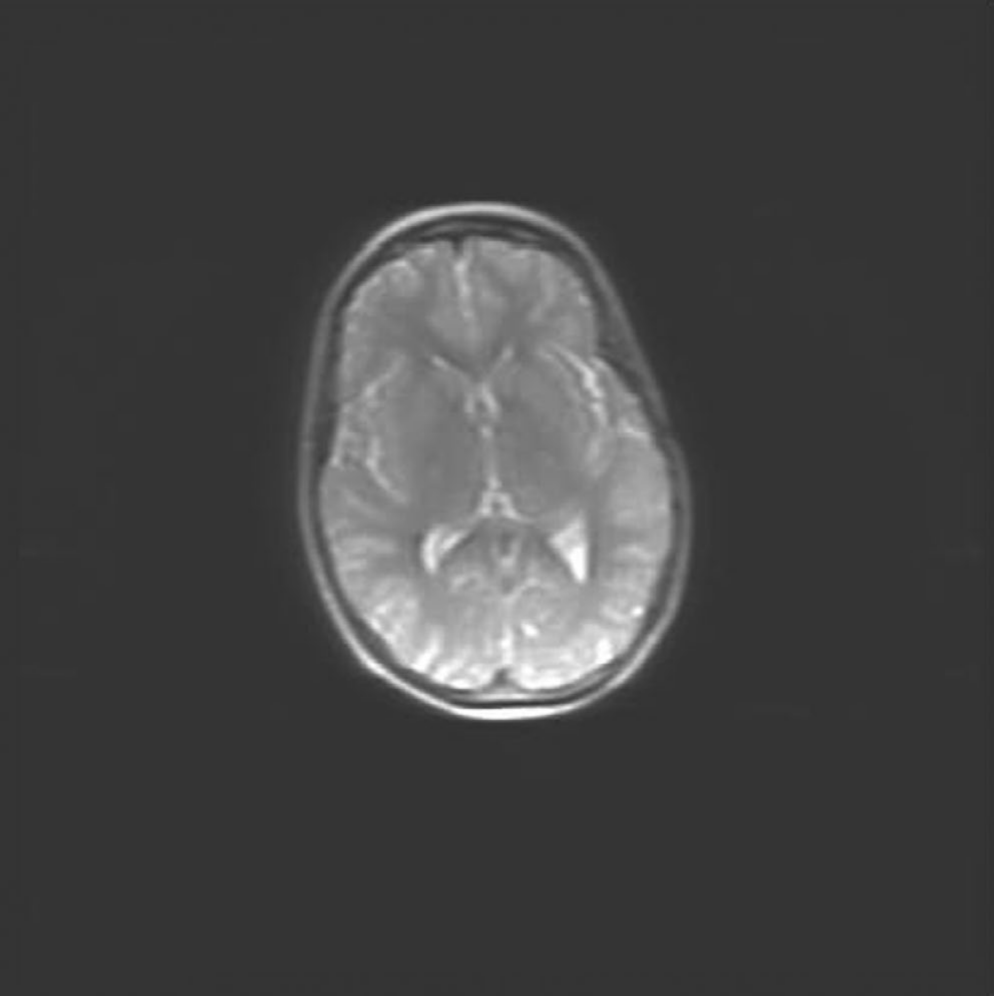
Magnetic resonance imaging at the end of MATRix (methotrexate, cytarabine, thiotepa, and rituximab) regimen (complete response)
